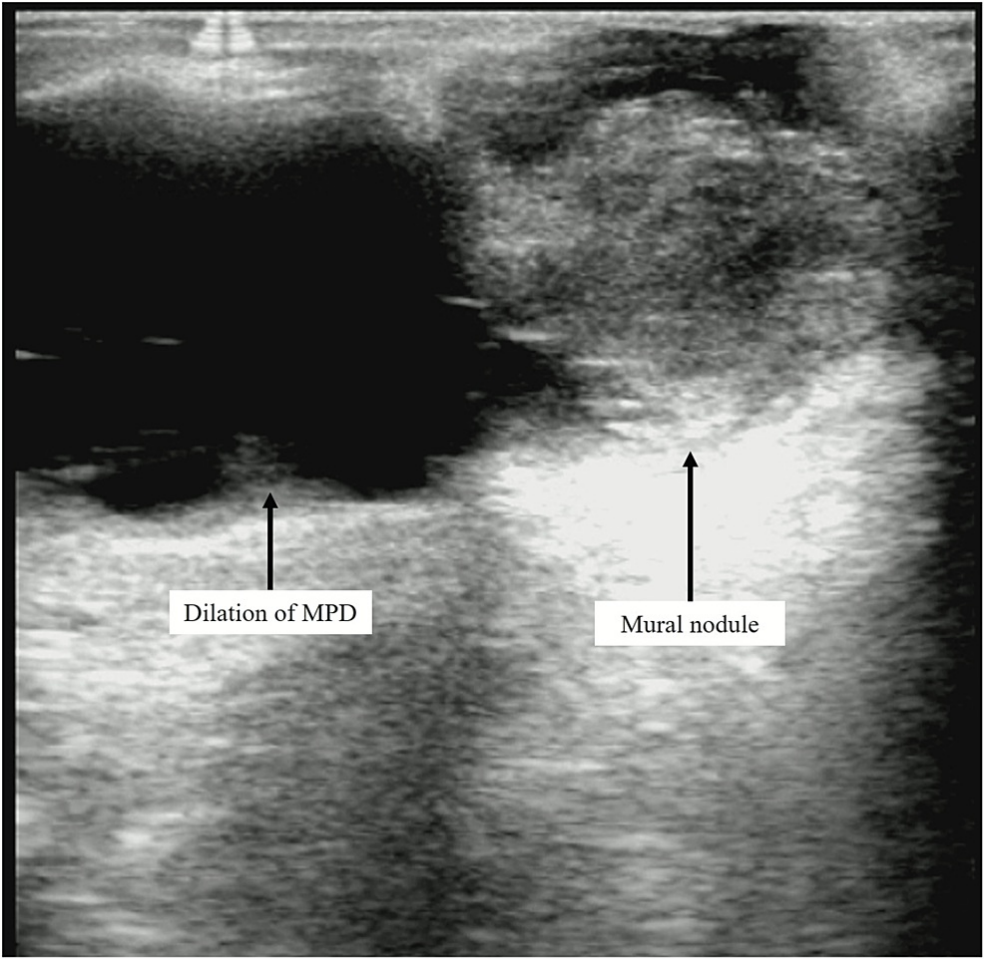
IOUS revealed a 14 mm mural nodule in the pancreatic tail with MPD dilation. Abbreviations: IOUS, intraoperative ultrasound; MPD, main pancreatic duct.
